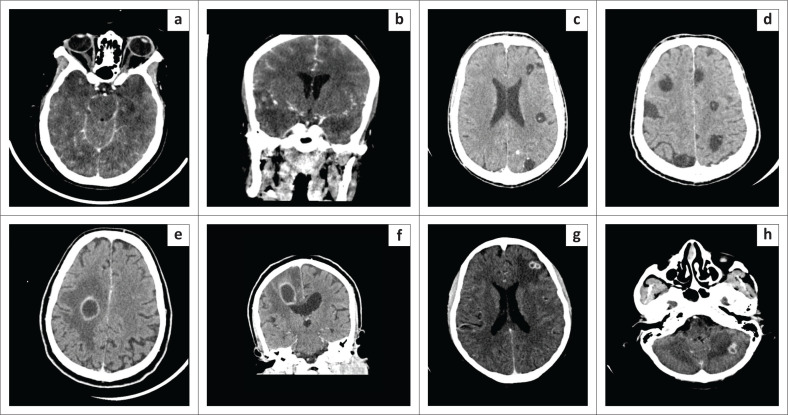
Infective pathology as a cause of adult-onset seizures. (a and b) Herpes Simplex (HSV) encephalitis in a young immunocompromised female patient. Axial NECT: Bilateral anterior temporal lobe oedema with hyperdense foci in keeping with microhaemorrhages. (c and d) Neurocysticercosis in a 47-year-old male with newly diagnosed HIV. Axial - contrast enhanced CT (CECT): Multiple bilateral non-enhancing round CSF density cystic intra-axial lesions of varying sizes with central hyperdense scolices (dot sign). (e and f) HIV positive patient on tuberculosis treatment. Coronal and axial CECT: Large right frontal lobe ring-enhancing lesion adjacent to the right lateral ventricle. Surrounding vasogenic oedema with associated mass effect. A differential diagnosis of a pyogenic or a tuberculous abscess was given. (g and h) A 53-year-old HIV positive male patient. CD4 = 26 cells/μL. Axial CECT: Multiple ring-enhancing lesions with vasogenic oedema in the cerebellum and corticomedullary junction of cerebrum. A differential of toxoplasmosis and tuberculomas was given.
